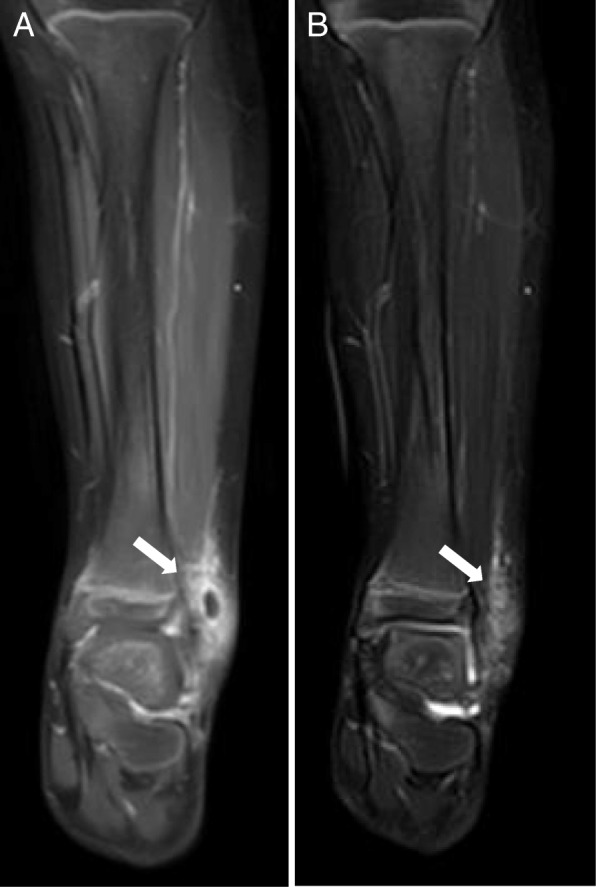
Magnetic resonance imaging of the left lower leg. T1 with enhancement (a) and T2-weighted images (b) on day 3 are shown. Note that hyper-intense signals (arrows) are present at the left lateral condyle
